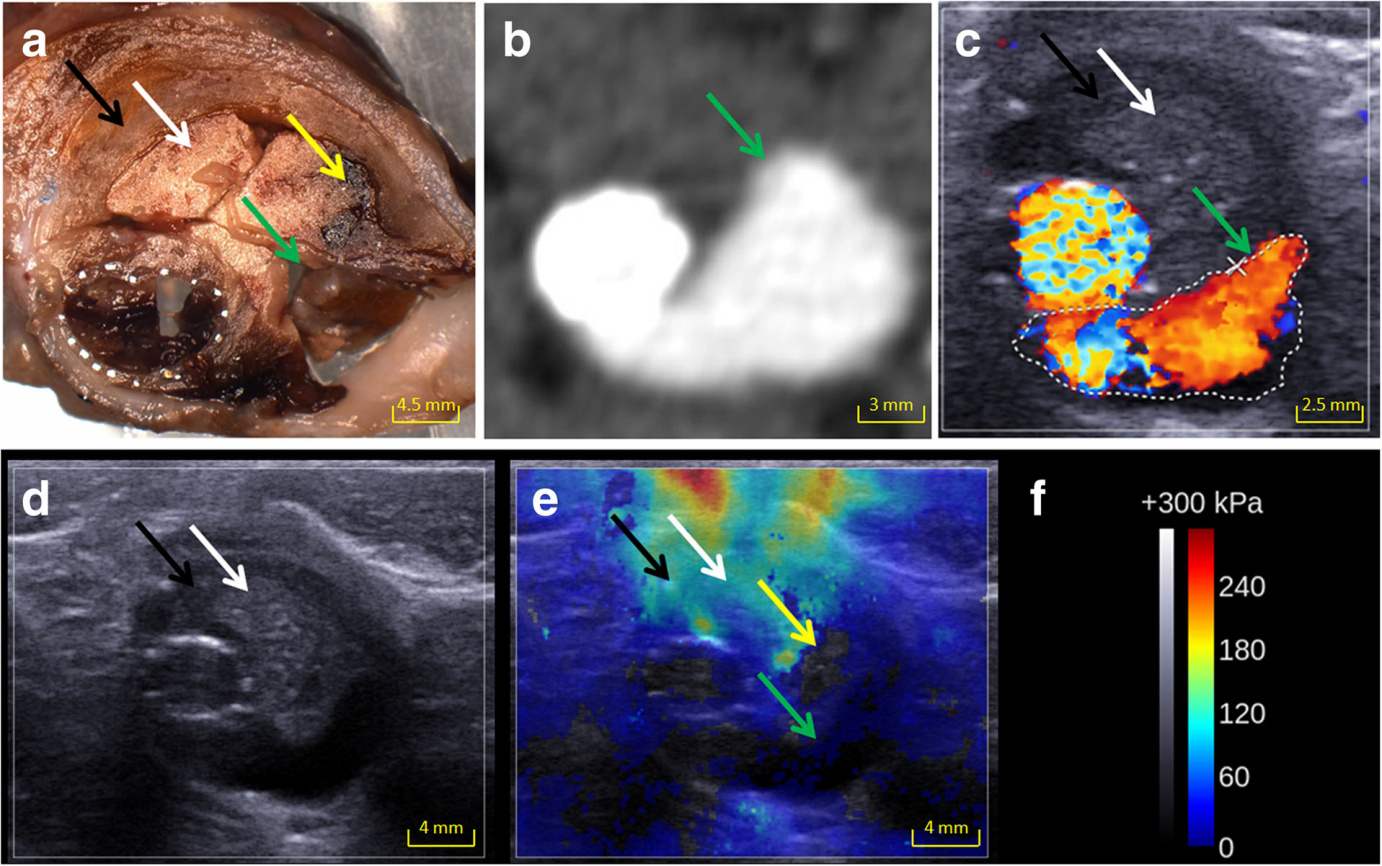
Images from an aneurysm with endoleak (*green arrow*), chitosan (*white arrow*), less-organised chitosan (*yellow arrow*) and organised thrombus (*black arrow*). a Macroscopic cut showing the different regions of interest. b CT scan showing the endoleak. c Endoleak on DUS. d Areas filled with chitosan were slightly hyperechoic and areas with endoleak were anechoic on B-mode ultrasound. e Endoleak and less organised chitosan were visualised on SW. f Colour scale and Q-Box values for SWI
